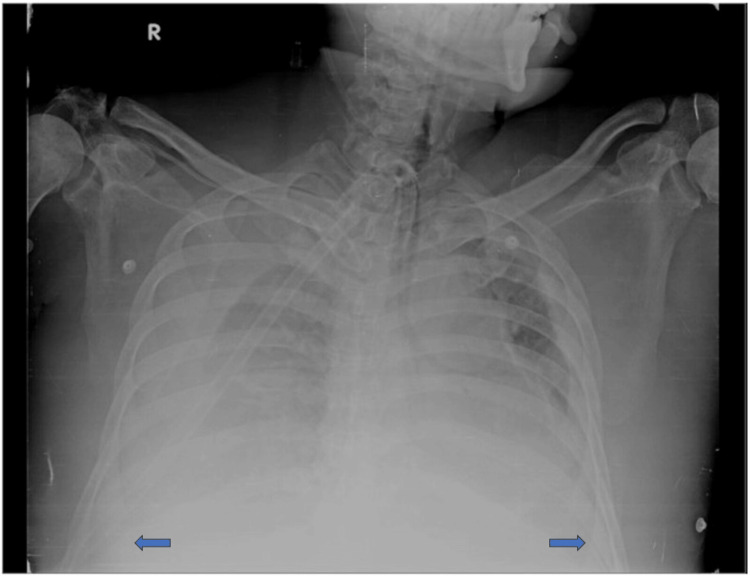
Chest X-ray PA view suggestive of significant bilateral pleural effusion (blue arrow).
